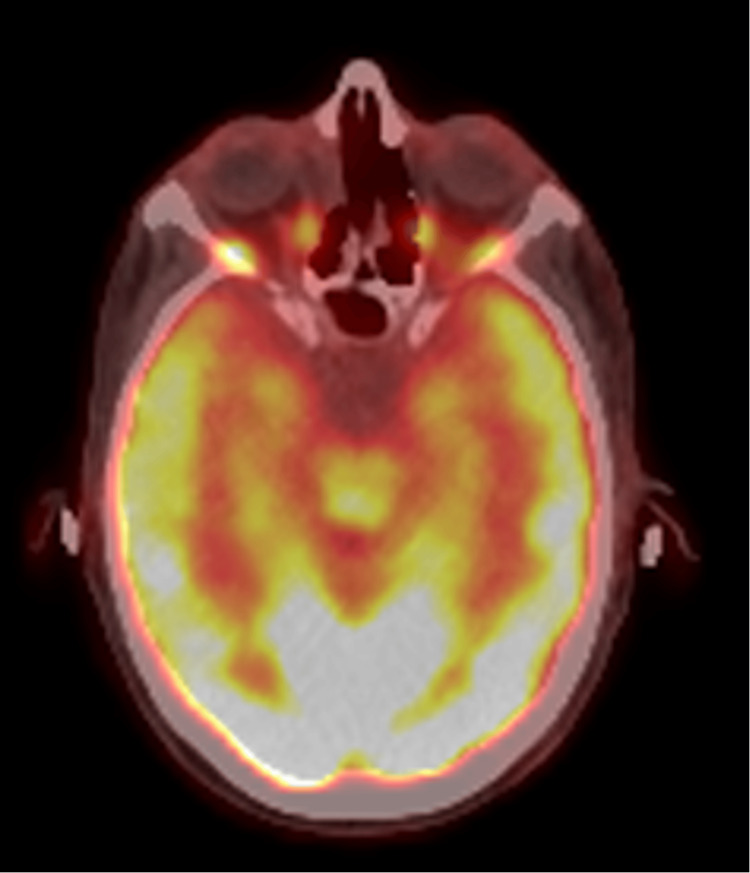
PET-CT from December 3, 2025. PET-CT: positron emission tomography-computed tomography
